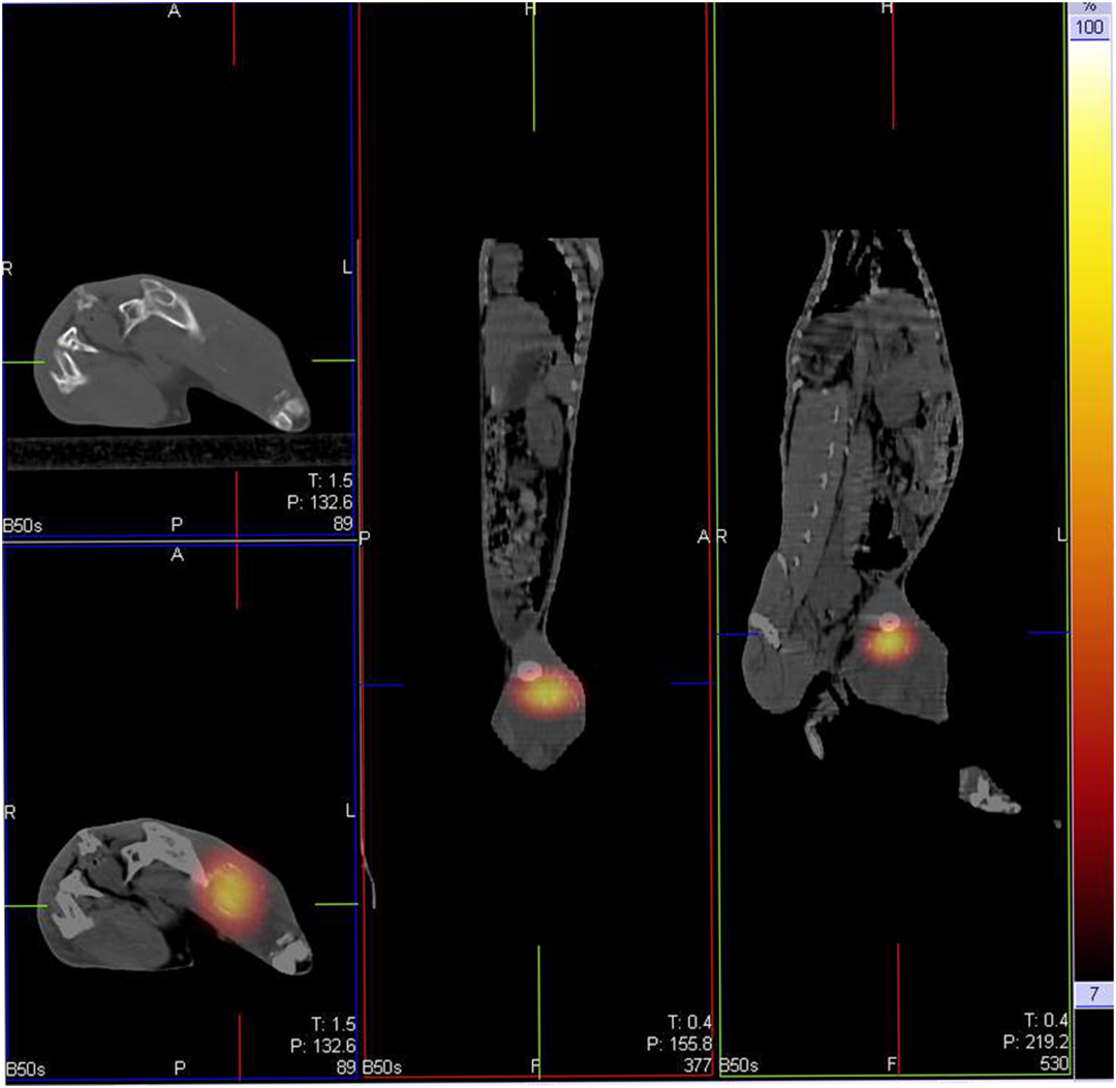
The SPECT/CT fusion images. The fusion images show the injected ^131^I-chTNT overlaps with the tumor.
